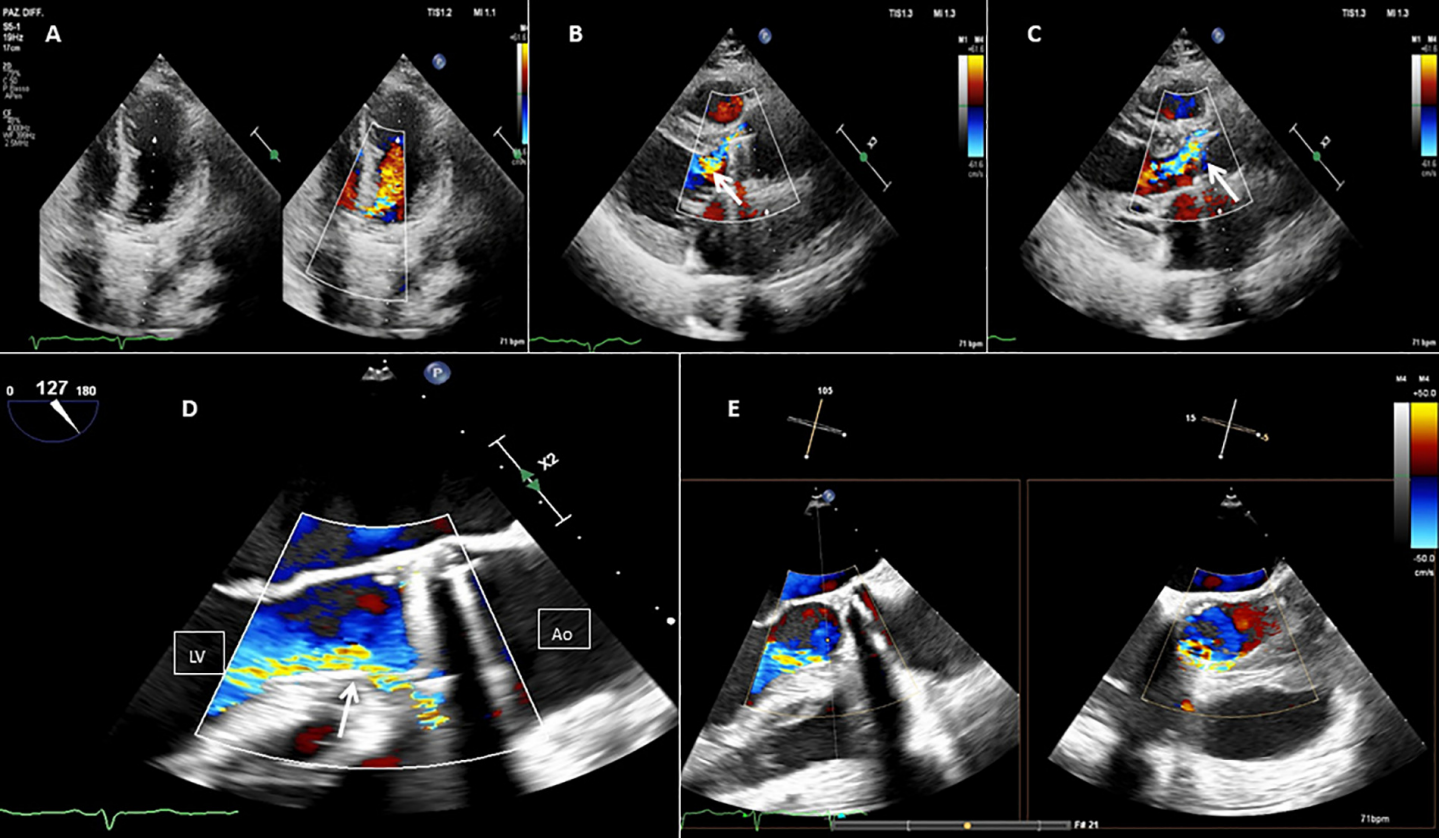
Baseline 2D TEE color Doppler at the apical five chamber view (A), at the parasternal long axis view (B,C), at the mid-esophageal (ME) long axis view (D), and at the ME long axis view with Xplane (E), showing an On-X® prosthetic aortic valve with moderate-to-severe regurgitation (orange arrow) through a long paravalvular leakage. Red line, PVL; LV, left ventricle; Ao, aortic valve.
